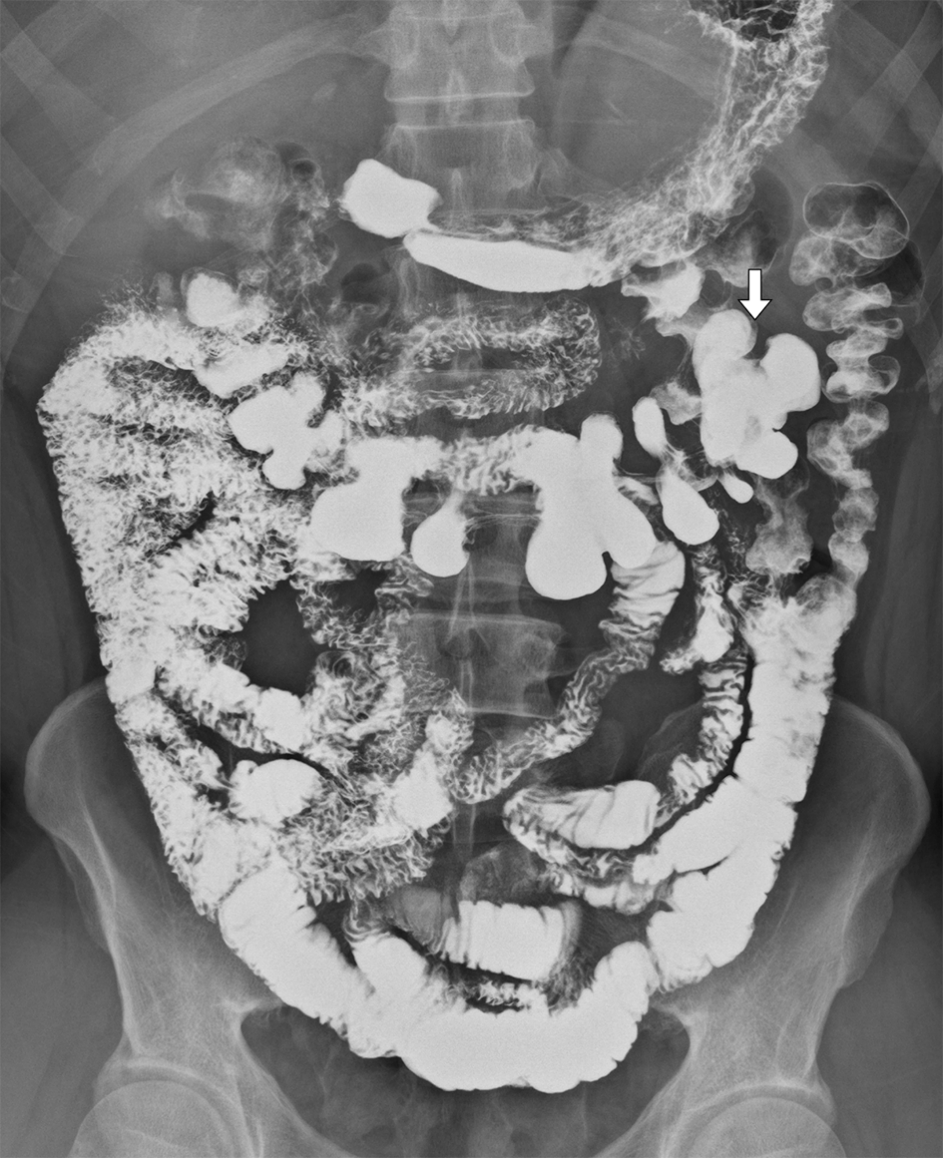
Small bowel GI series using X-ray contrast media. The cecum (arrow) and proximal ascending colon are located in the LUQ of the abdomen, and most of the small intestine is distributed in the right lateral abdomen.
